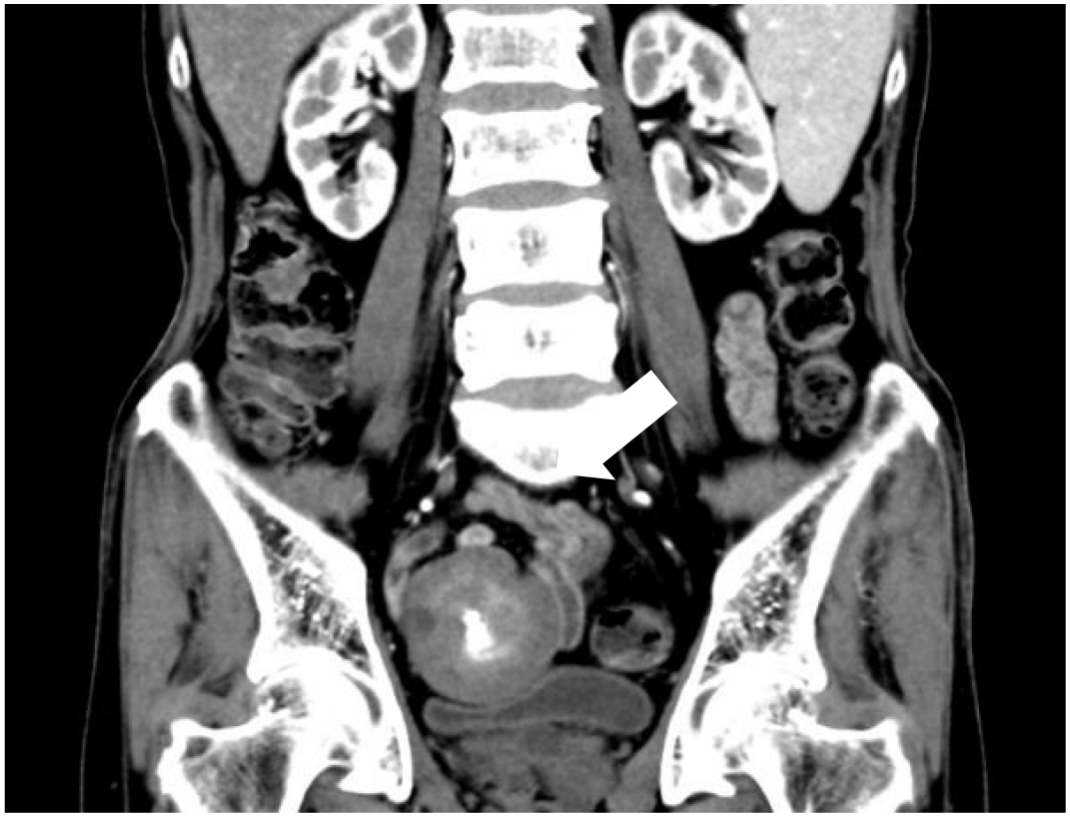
Computed tomography shows that the mass (indicated by the white arrow) demonstrates no enhancement and is located in the pelvis, compressing the bladder.
